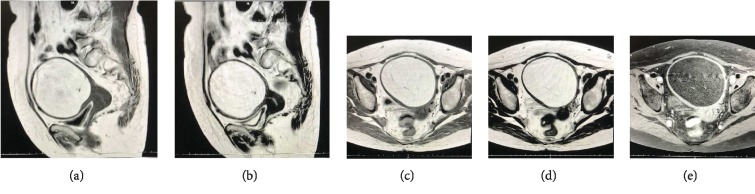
Sagittal (a, b) and axial (c–e) MRI images of the uterine lipoma showing high signal on T1 (a, c) and T2 (b, d) sequences and low signal on fat suppression sequence (e).
